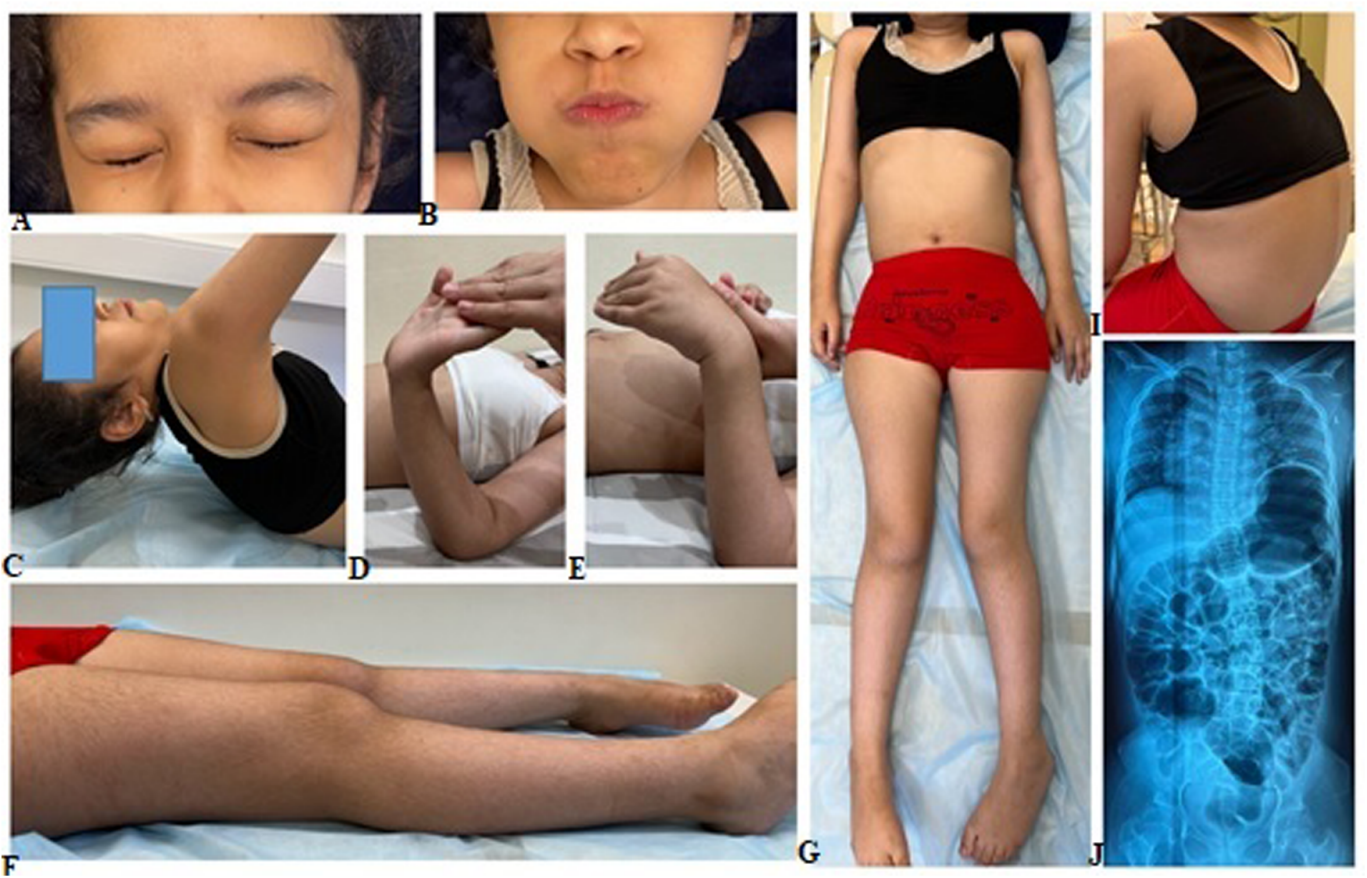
Clinical manifestation of the proband's sister included pronounced kyphoscoliosis, hypermobility of distal phalanges, varus deformity of the feet, shorter left leg, generalized hypotonia, and severe weakness in the muscles of the neck and lower limbs (A–I). X-rays of the spine and pelvis shows left-sided lumbar scoliosis and subluxation of the femoral (J).
